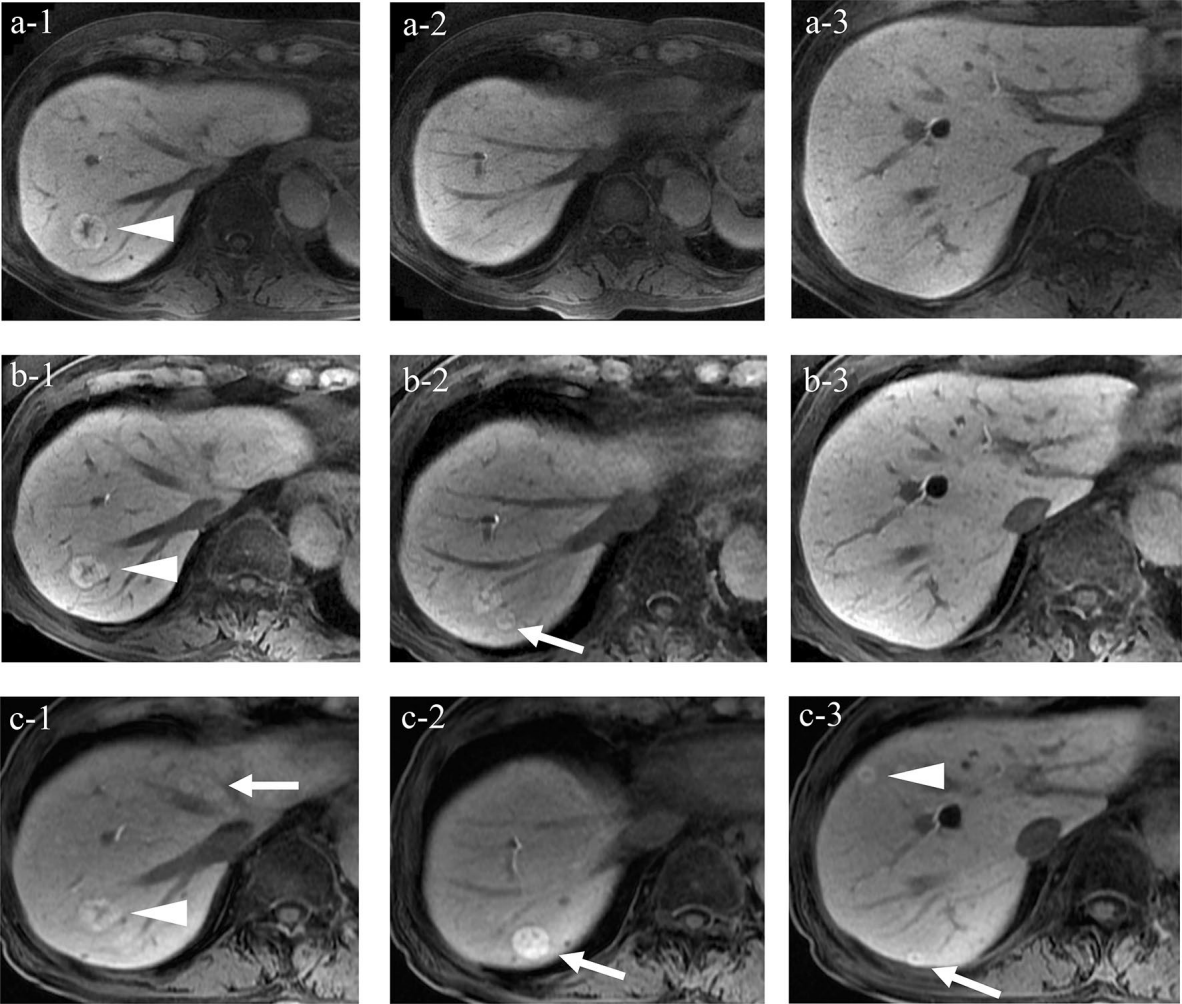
The changes in the EOB-MRI hepatobiliary phase findings over time. a-1 At 79 years of age: a 25 mm nodule with clear borders was noted in segment 7 region with a hypointense center and a hyperintense surrounding area (arrowhead). a-2 No mass was noted behind the nodule in segment 7. a-3 No mass was noted in segments 7 or 8. b-1 At 87 years of age: the nodule in segment 7 showed no major change (arrowhead). b-2 A 12-mm nodule exhibiting similar findings was noted behind the nodule in segment 7 (arrow). b-3 No mass was noted in segments 7 and 8. c-1 At 89 years of age: the nodule in segment 7 showed no major change (arrowhead) and a 10-mm nodule appeared in segment 4 (arrow). c-2 The nodule behind the nodule in segment 7 increased to 20 mm (arrow). c-3 Nodules of 10 mm in diameter appeared in segments 7 (arrow) and 8 (arrowhead). *EOB-MRI* gadolinium-ethoxybenzyl-diethylenetriaminpentaacetic acid-enhanced magnetic resonance imaging
